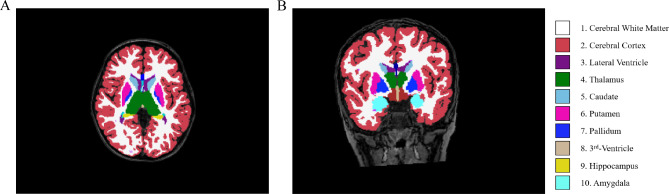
Segmentation of the subcortical structures. 3D T1-weighted image of a participant with delineation of the subcortical structures: cerebral white matter (color 1), cerebral cortex (color 2), lateral ventricle (color 3), thalamus (color 4), caudate(color 5), putamen (color 6), pallidum (color 7), 3rd -Ventricle (color 8), hippocampus (color 9) and amygdala(color 10) in the axial (A) and coronal (B) planes
